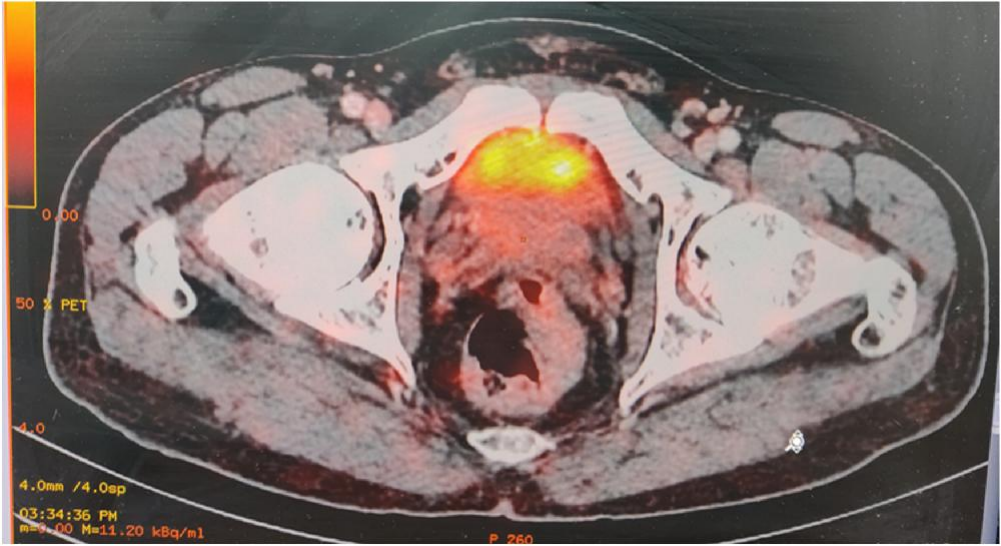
Post radiofrequency ablation, Ga 68 DOTANOC PET/CT scan showing the absence of uptake in the previously involved area.
